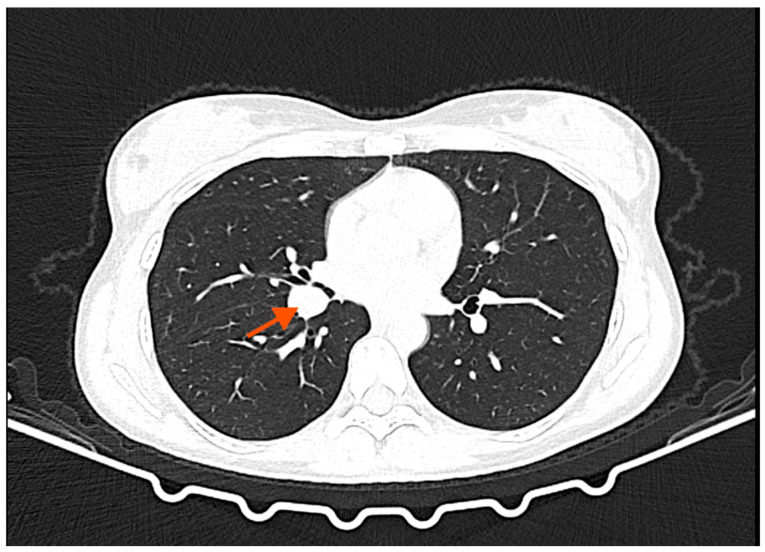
A 30-year-old female, non-smoker, with no known occupational exposures or significant comorbidities, was referred for pulmonology evaluation due to recurrent episodes of stridor and wheezing. Initially nocturnal, the symptoms progressively increased in frequency and began occurring during daytime, with notable exacerbation over the last two months. The patient denied fever, hemoptysis, weight loss, or other systemic symptoms. Notably, the symptoms had been present for approximately two years and had been attributed to asthma, for which the patient had received bronchodilator therapy without meaningful improvement—a common pattern of diagnostic delay in endobronchial neoplasms. Such a clinical presentation is consistent with the broad and often nonspecific respiratory manifestations described in bronchial carcinoid tumors, which may include wheezing, cough, dyspnea, or recurrent infections, although a subset of patients may remain asymptomatic [1]. Physical examination revealed a patient in good general condition, afebrile, with normal cardiovascular findings. Respiratory auscultation demonstrated expiratory wheezing, consistent with the symptomatic presentation, while no signs of respiratory distress or accessory muscle use were observed at rest. Routine laboratory investigations were within normal limits, except for a mild indirect hyperbilirubinemia of no clinical significance. Pulmonary function testing, including spirometry, plethysmography, and diffusion capacity, demonstrated values within predicted ranges, with no significant bronchodilator response (FVC 3.21 L [88%], FEV1 2.49 L [79%], FEV1/FVC 77.41%, PEF 4.31 L/s [61%], MEF-50 84.88%, DLCOc 82%, KCOc 94%). Notably, the peak expiratory flow was disproportionately reduced relative to FEV1 (PEF 61% vs. FEV1 79% predicted), a subtle spirometric asymmetry that, in retrospect, may reflect the influence of the central airway lesion on peak flow generation. The Empey index (FEV1[mL]/PEF[L/min]), calculated from these values, yielded 9.63—approaching but not exceeding the classical threshold of 10 for fixed upper airway obstruction, consistent with a lesion situated below the glottic level, where the index has reduced sensitivity compared to subglottic or tracheal pathology [2]. Despite the presence of wheezing and stridor, the absence of functional impairment suggested a localized obstructive process not detectable by standard pulmonary function testing, raising suspicion for a central airway lesion. Contrast-enhanced thoracic CT identified a well-circumscribed, moderately enhancing perihilar lesion in the right lung, measuring approximately 15 × 13 mm in the transaxial plane, situated adjacent to the division of the right pulmonary artery—anatomically corresponding to the level of the distal bronchus intermedius, just proximal to the origin of the right lower lobe bronchus. No mediastinal or hilar lymphadenopathy was observed, and no pleural or pericardial effusion was present. This pre-procedural CT characterization was of paramount importance in planning bronchoscopy, as it anticipated the critical relationship between the lesion and the right middle lobe carina. The red arrow indicates the perihilar right-sided endobronchial lesion adjacent to the right pulmonary artery. In this context, computed tomography plays a crucial role, as it typically demonstrates well-defined endobronchial or perihilar lesions with a round or ovoid configuration, sometimes associated with calcifications [3]. The imaging appearance raised the suspicion of an endobronchial tumor, with differential diagnoses including carcinoid tumor, bronchogenic carcinoma, or less likely benign lesions such as hamartoma.
